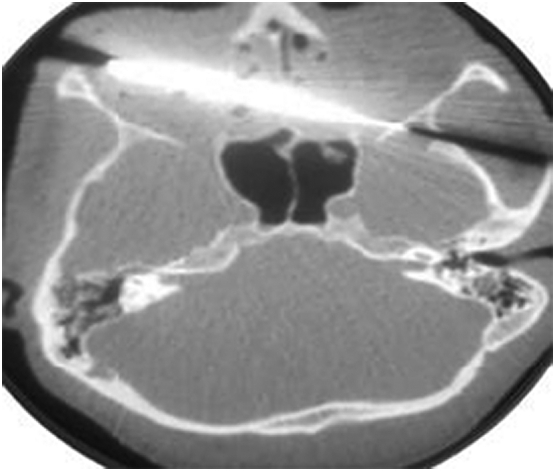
Axial CT-scan showing the knife shattering the right eyeball and severing the left optic nerve.
